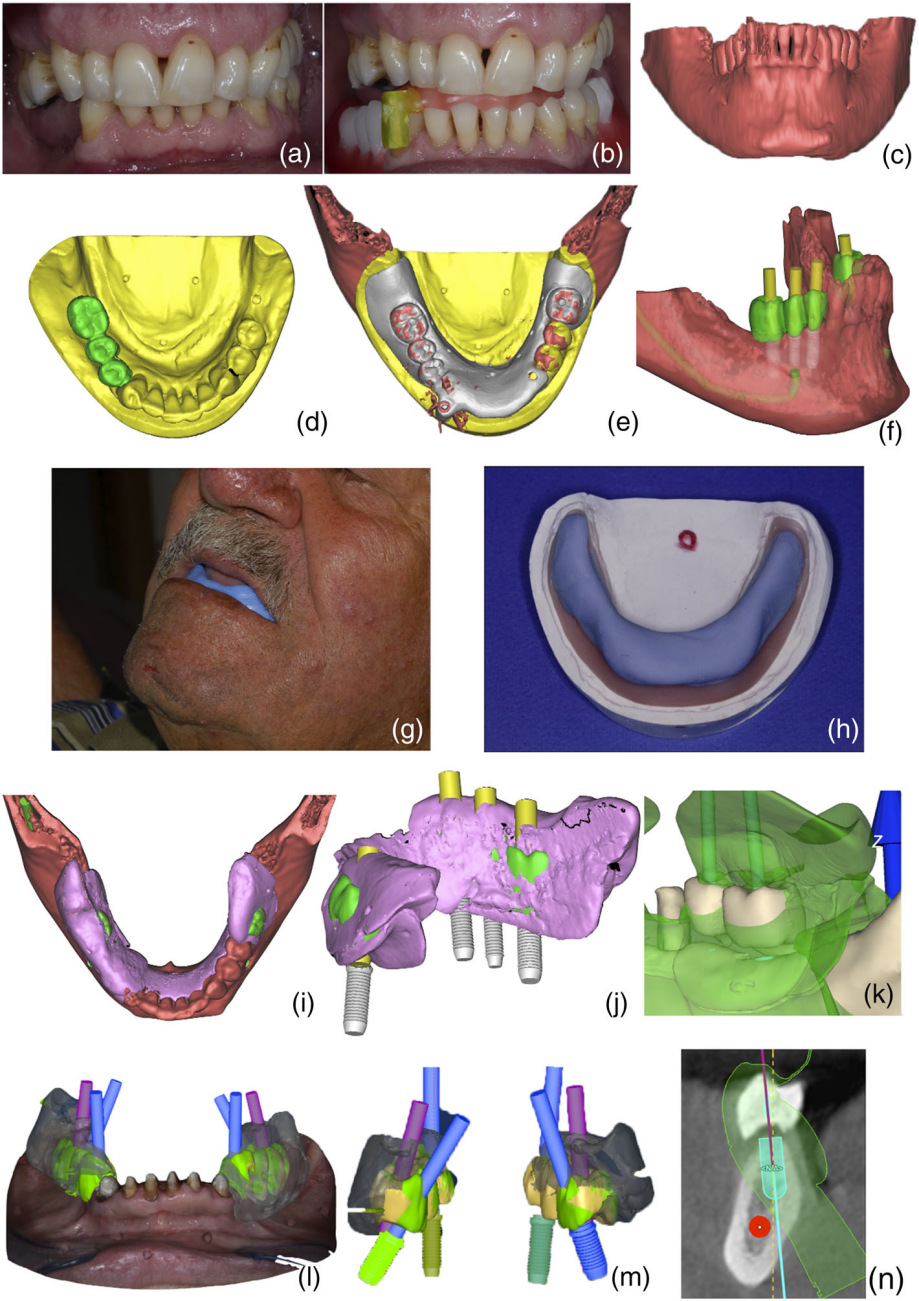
Study procedures were summarized in those panels. (a) Patient involved in the study. The tongue overrun edentulous space. (b) Radiographic guide check on edentulous arch. (c) Cone beam–computed tomography 3D rendering. (d) Optical scan data. (e) Integration of surface anatomical data, from surface scanner, and 3D radiographic data. (f) Computer and prosthetic guided implant planning. (g) Neutral zone (NZ) registration procedures. (h) Piezographic record on its model. (i) NZ registration integrated in the virtual environment using the model cast as reference. (j) Implant planning based on piezographic data. (k) Traditional teeth arrangement does not respect NZ on the lingual side. (l,m) Differences of implant axes planned on traditional teeth arrangement technique (in blue) and on piezographic data (in violet). (n) Piezography‐based implant planning in cross‐sectional view. The software generates angular measurement referring to the dotted line, which is perpendicular to occlusal plane. Note the invasion of conflict zone by tooth of radiographic template
